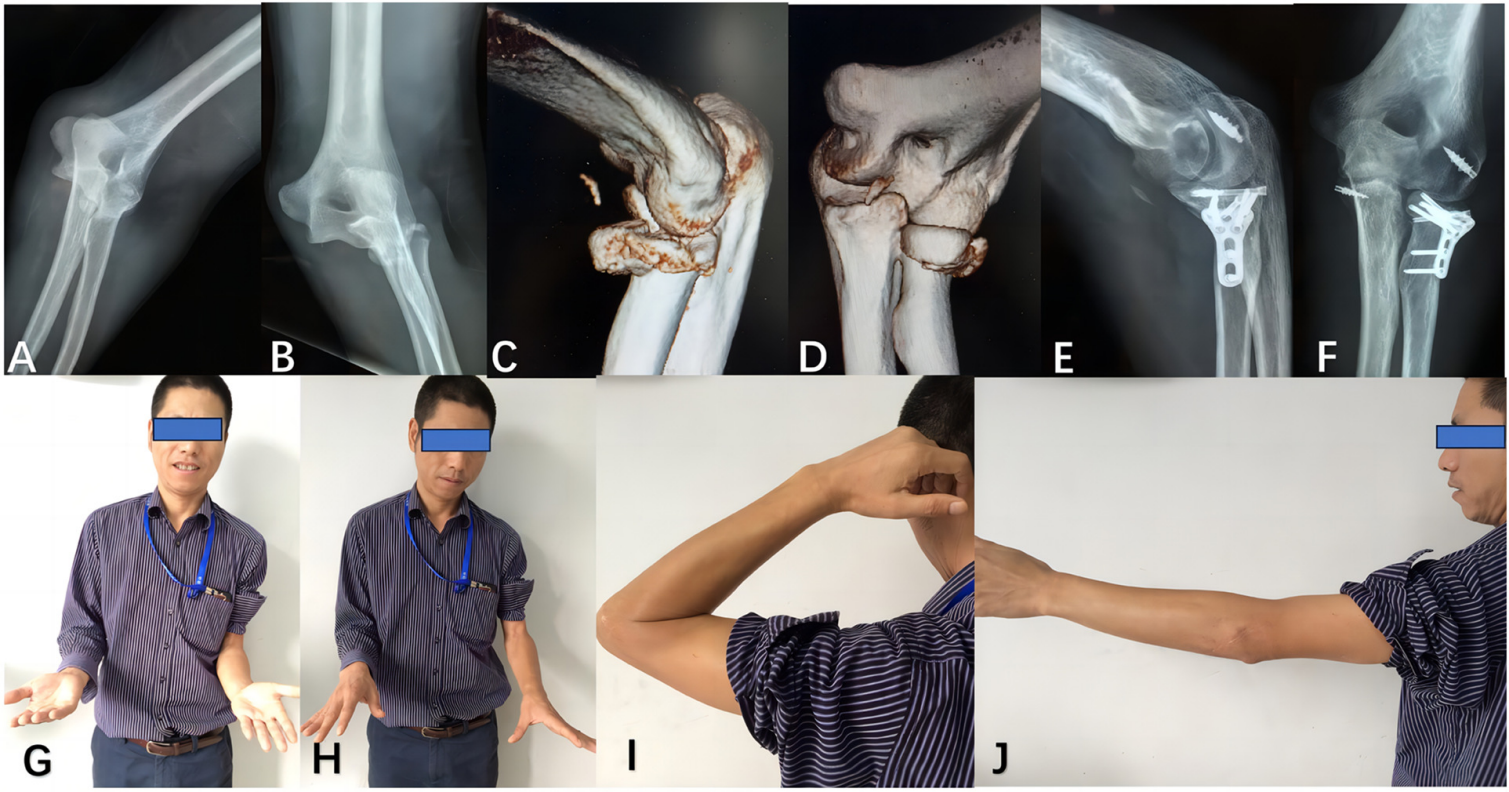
The patient underwent treatment for a terrible triad injury, consisting of a radial head fracture, concomitant elbow dislocation, and coronoid process fracture, using both lateral and medial approaches. Preoperative anteroposterior and lateral radiographs (A,B), as well as computed tomography images (C,D), revealed a Mason-Johnston type IV radial head fracture with associated coronoid process fracture and elbow dislocation. Postoperative radiographs (E,F) were obtained following osteosynthesis of the radial head and reconstruction of the lateral and medial collateral ligaments. At the last follow-up (G–J), satisfactory restoration of elbow function was achieved.
